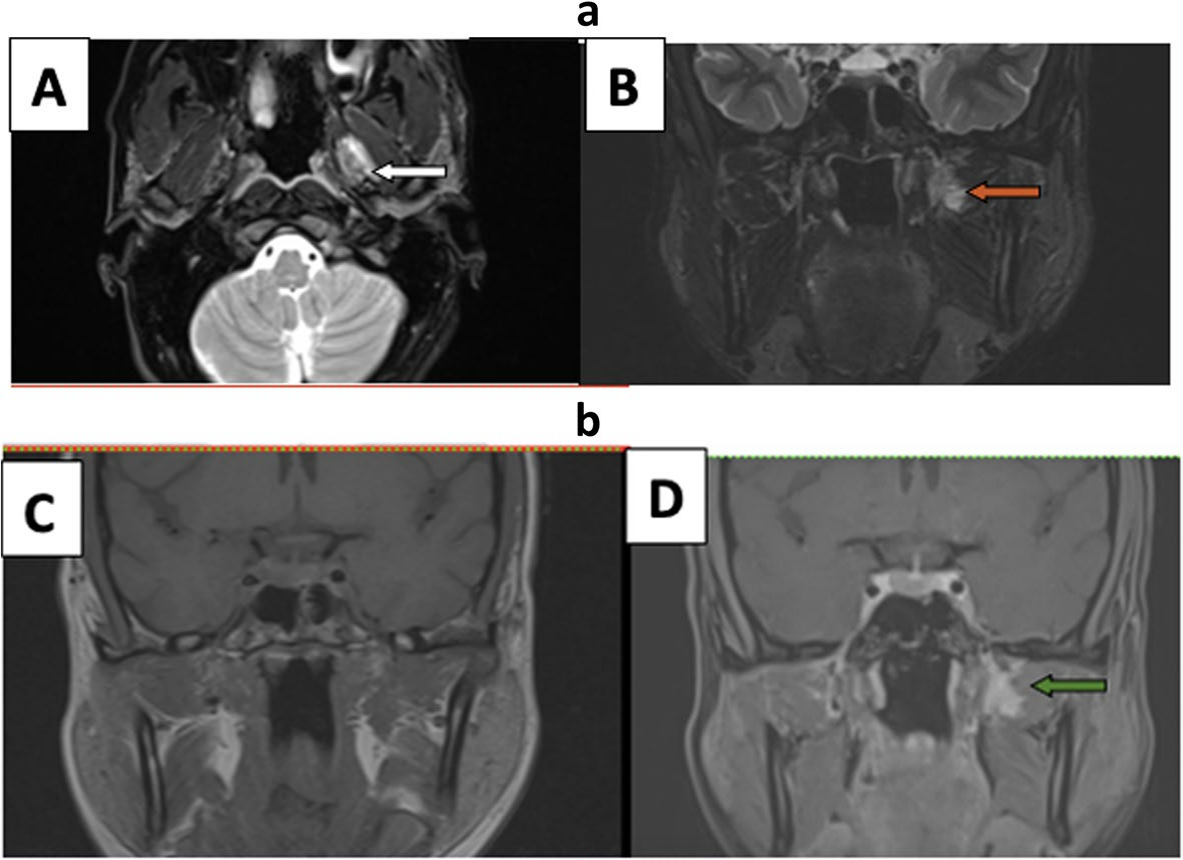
a (A, B) This patient with asymmetric ethmoidal and maxillary sinusitis (L>R) shows hyperintense lesion in left masticator space on axial and coronal T2 fat-sat images (white and red arrows), also note there is a normal area between posterior aspect of maxillary sinus and lesion with no involvement of retroantral fat. b (C, D) Plain and post-contrast fat sat T1-weighted images confirmed lesion to be hemangioma in view of avid enhancement (green arrow) of lesion
